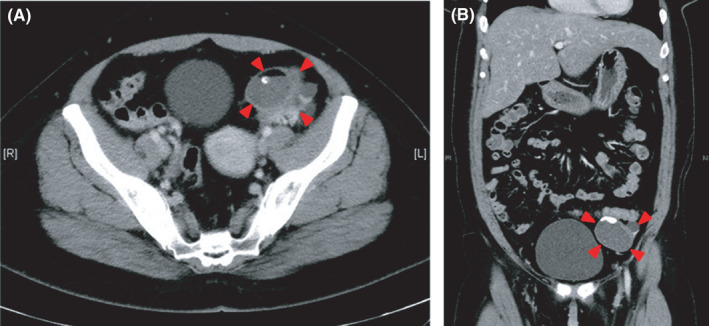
Computed tomography imaging findings. (A) Transverse computed tomography (CT) and (B) coronal CT images demonstrate 55‐mm cystic lesions with calcification and fluid formation in the left ovary (red arrowheads). The presence of torsion is unknown
